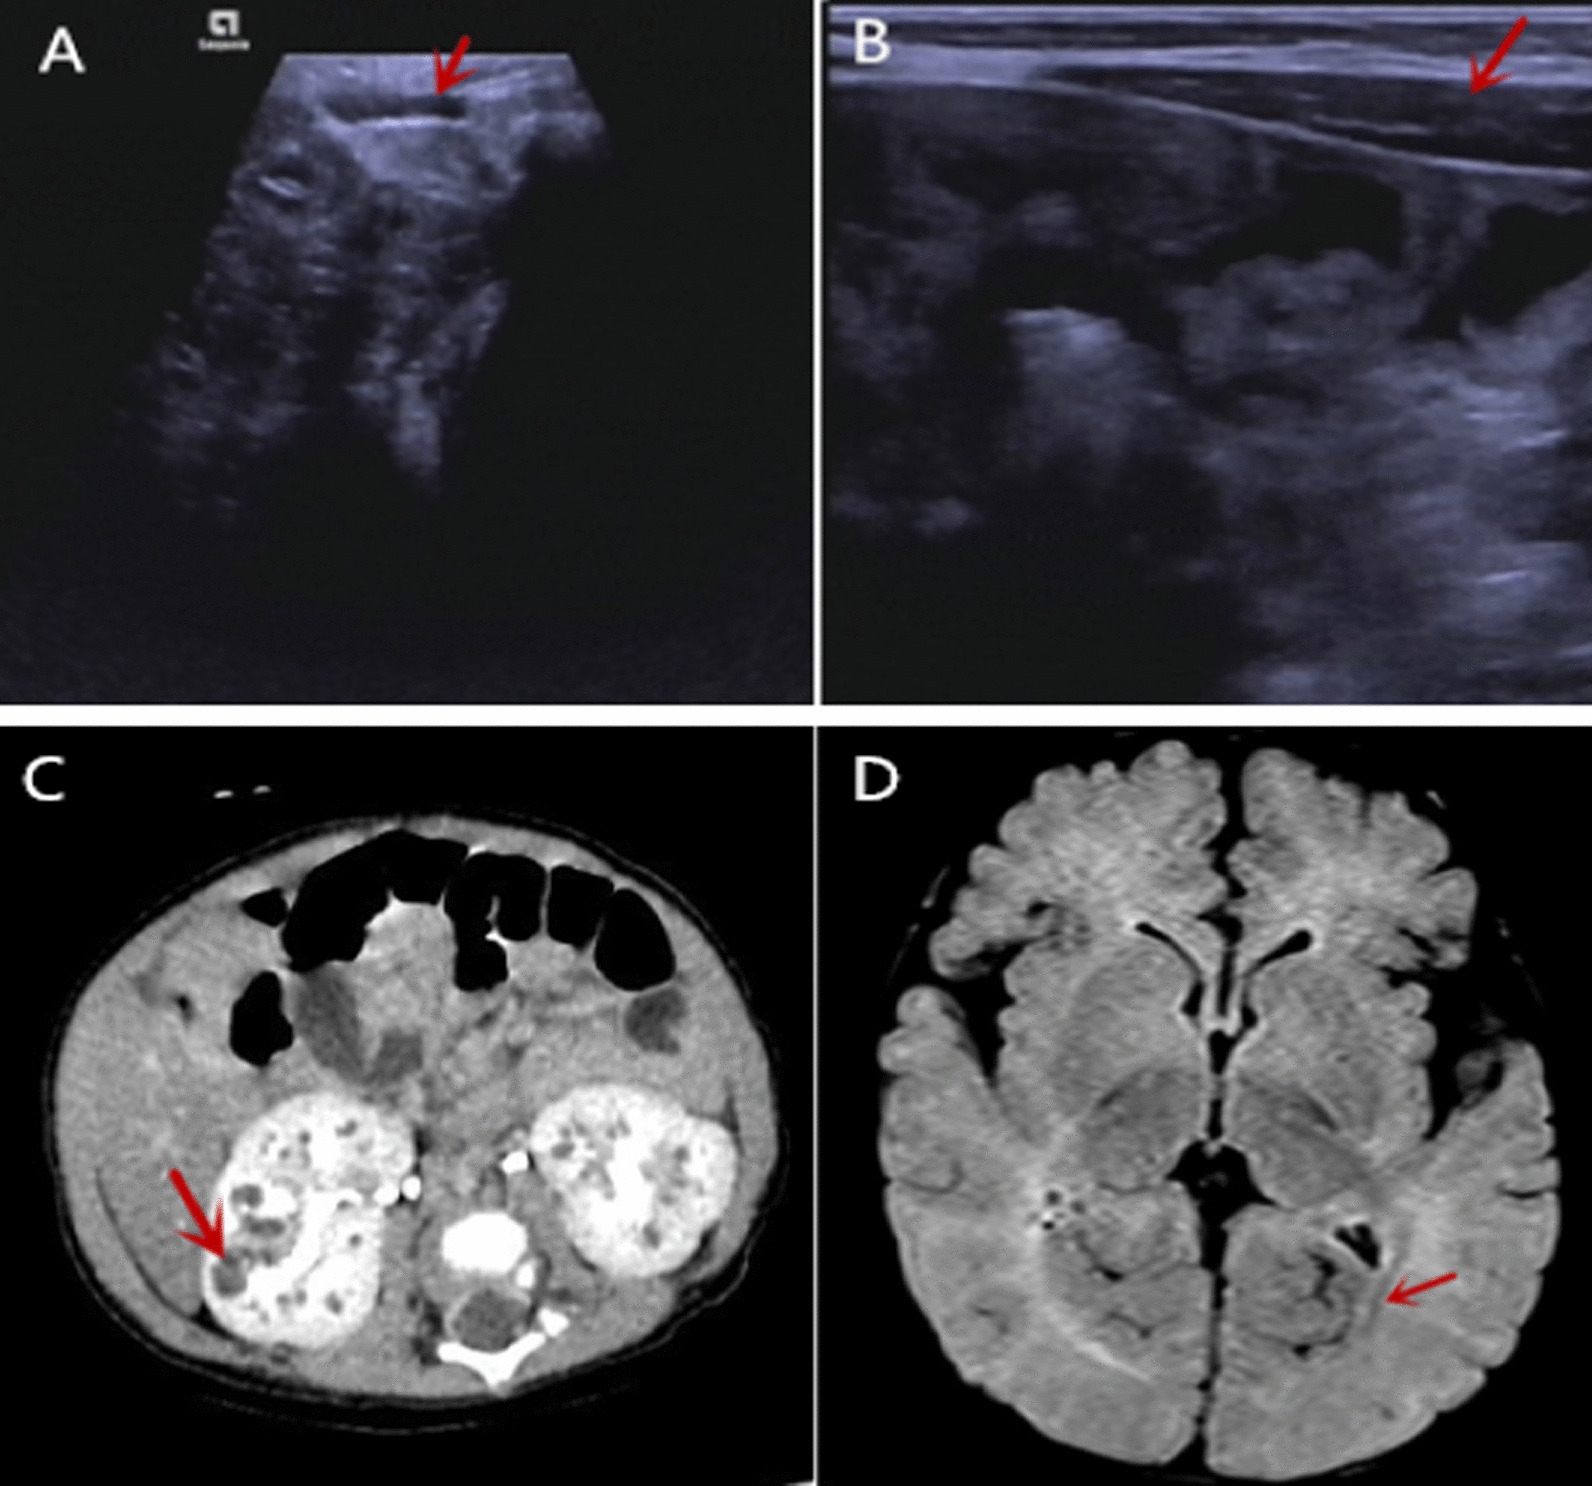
Umbilical sinus, polycystic kidney, and myelination dysplasia of the patient. A, B Umbilical color Doppler ultrasound showed abnormal echo (red arrow) in the umbilical area. C Abdominal CT showed that the volume of both kidneys was increased, and multiple small sac-like unenhanced areas of different sizes were detected (red arrow) in the renal medulla. D Cranial MRI showed the left cisterna and the anterior temporal space were slightly widened, and the posterior horn of bilateral ventricles manifested small patchy abnormal signal foci (red arrow)
